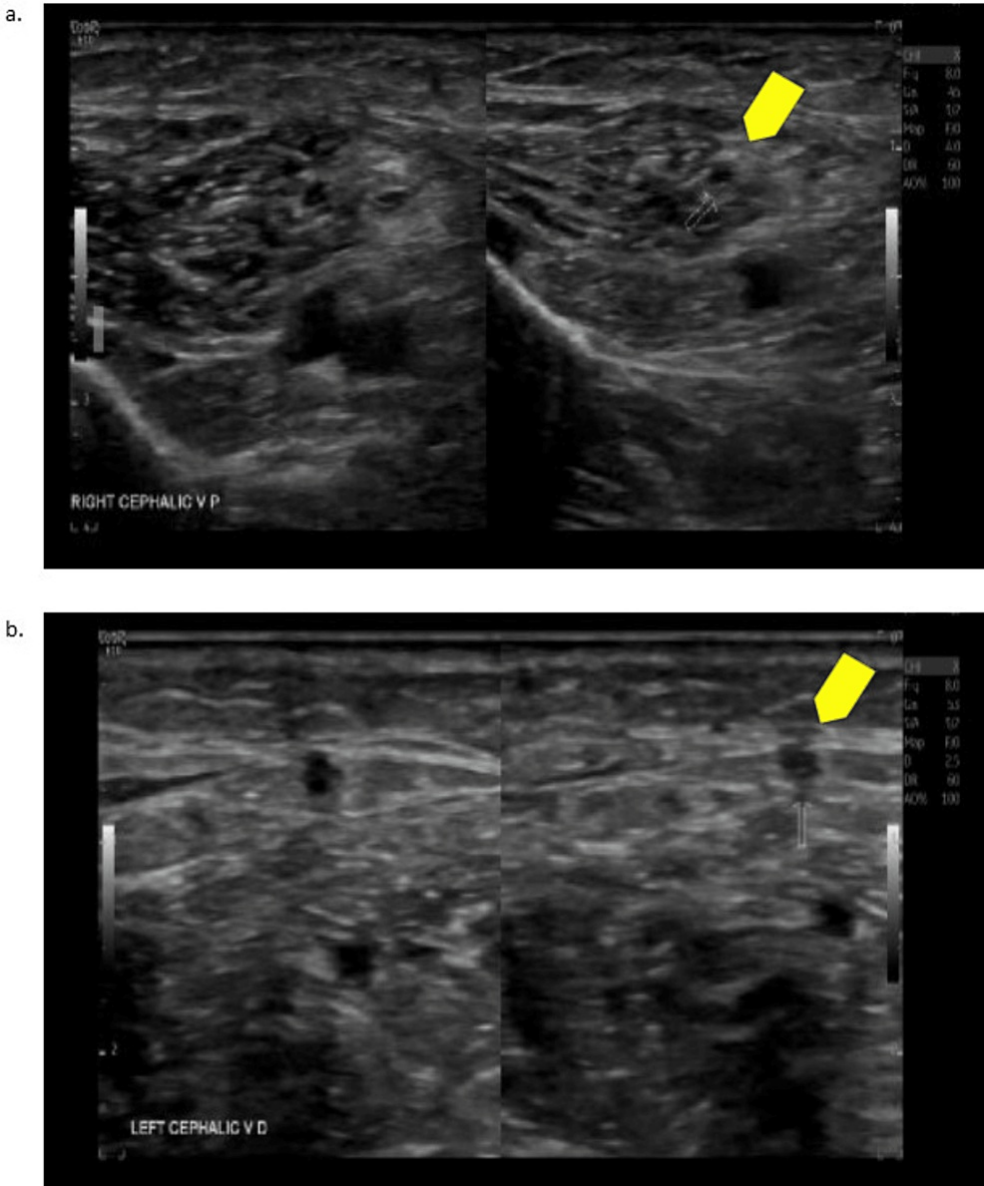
Ultrasounds of Bilateral Cephalic Veins a: right cephalic vein (yellow arrow indicates thrombus), b: left cephalic vein (yellow arrow indicates thrombus)
